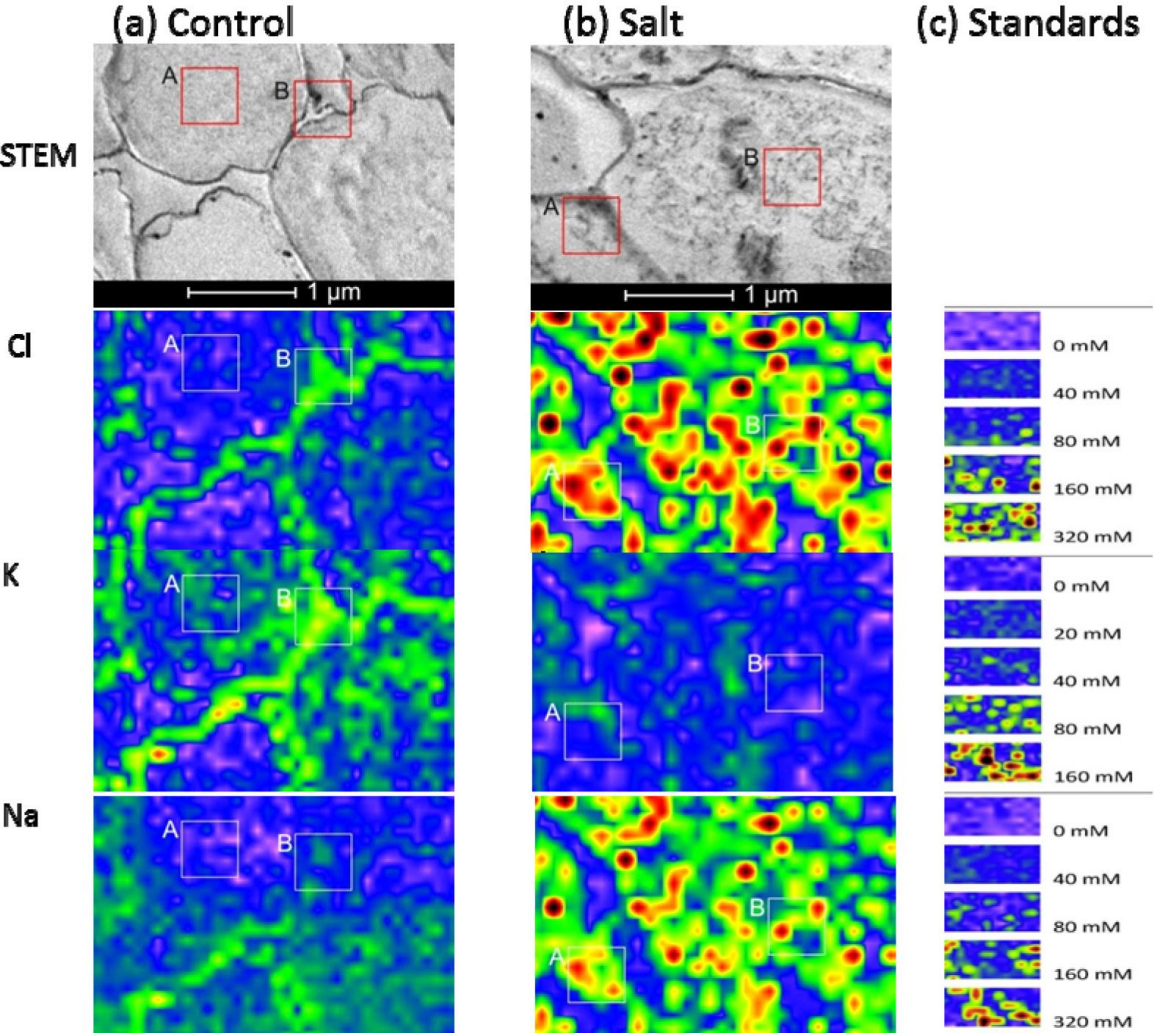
Representative STEM images and elemental images of root cortex in control (a) and NaCl-treated *P. euphratica* (b). For X-ray imaging, the area measured in root cortical cells depended on the cell structures of interest. The spatial resolution ranged from 50 nm × 50 nm to 100 nm × 100 nm. Elemental images of 5% agar standards (c) were acquired at the same resolutions. The maxima were set to 1000 for Na^+^ and Cl^−^ and 500 for K^+^; the minima were set to 30 for Na^+^, 25 for Cl^−^, and 10 for K^+^. Frames A and B were used to calculate the mean intensities (Table 1).
